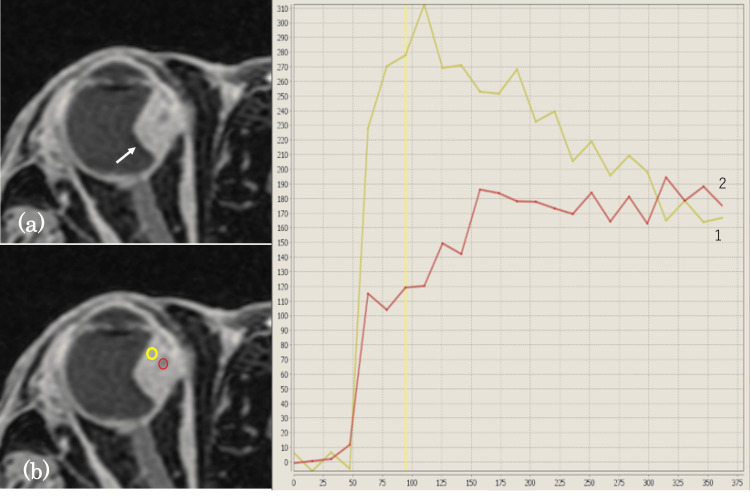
Axial DCE-MRI (a) and TICs (b) Axial DCE MRI (a) reveals that the mass has a heterogeneous enhancement (arrow). The TICs (b) are characterized by two types: early enhancement and washout (1) and persistent enhancement (2). 1 = margin of the mass (yellow ROI), 2 = center of the mass (red ROI). DCE-MRI: dynamic contrast-enhanced magnetic resonance imaging; TICs: time-intensity curves; ROI: region of interest
